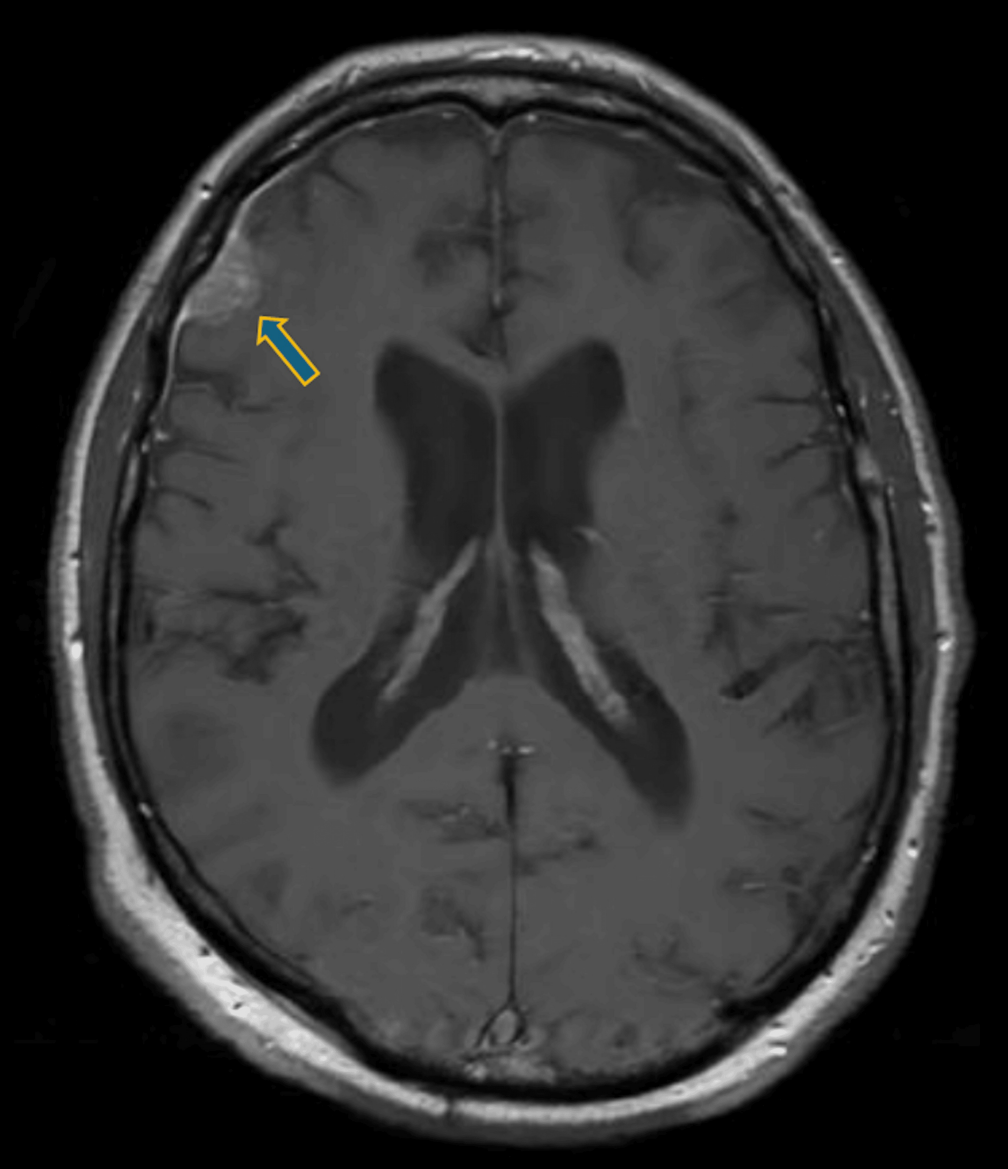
Brain MRI (T1 axillar) with contrast showed a right frontal lobe meningioma and enlarged ventricles.
